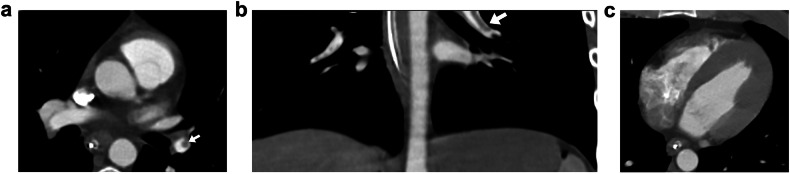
Panel graph outline. Example of a 31-year-old male soldier with a gunshot wound who was treated with an IVC filter following identification of pulmonary arterial clots on the CT. The initial assessment included an abdominal trauma CT protocol covering only the lower chest. a, b Axial and coronal views show a filling defect within the left lower lobar artery consistent with a pulmonary thrombus (PT) (arrows). c Axial view of the heart: the right to left ventricular ratio is normal
